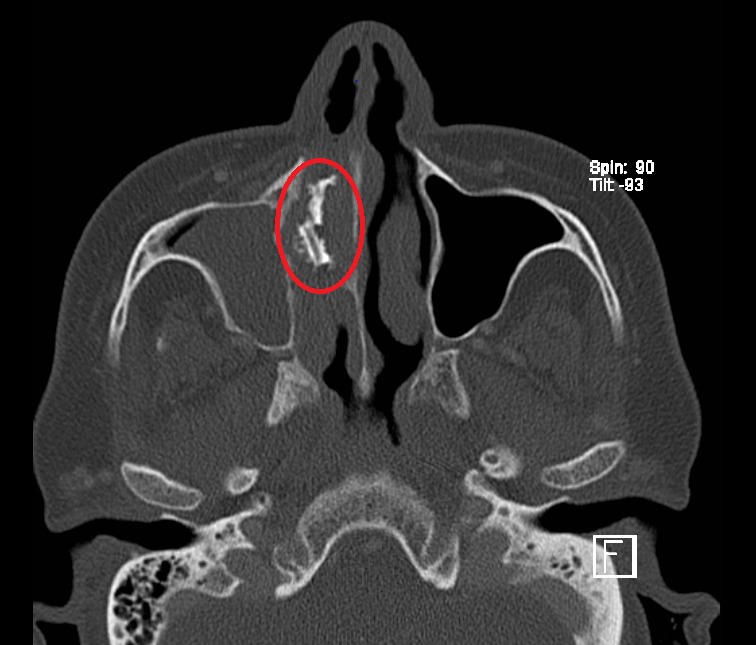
Preoperative axial CT imaging of paranasal sinus demonstrating a fragmented high‐density lesion within the underlying dacryocystocele. (circle).
